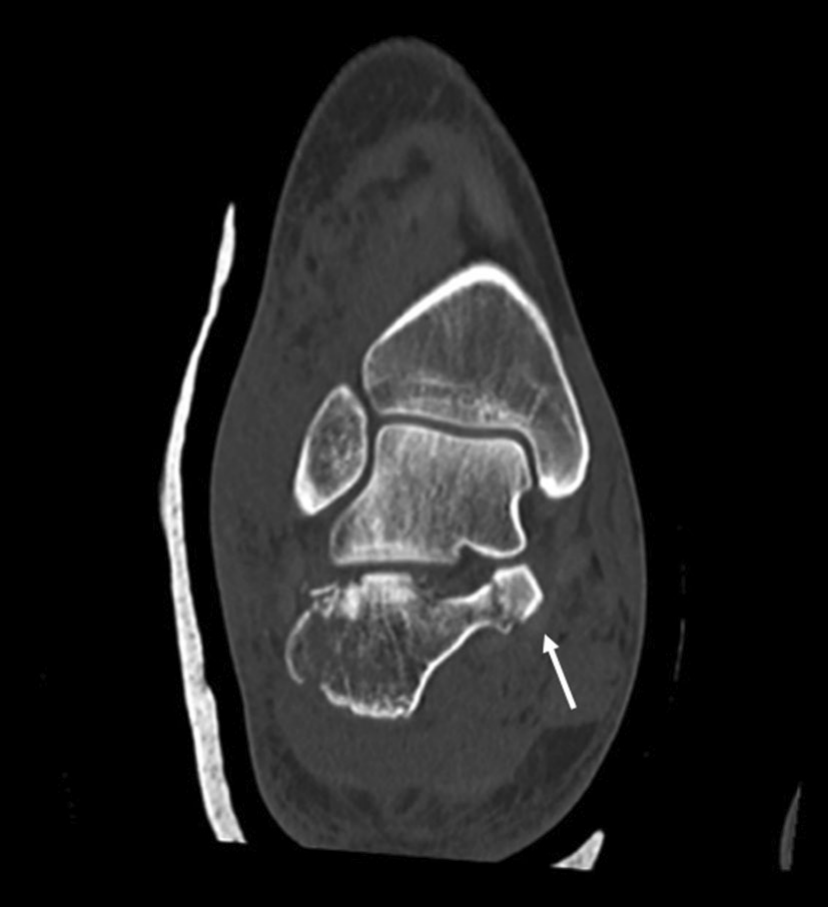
Semicoronal CT section. Fracture of the calcaneus. The white arrow shows the extension of the fracture line to the body of the sustentaculum tali
